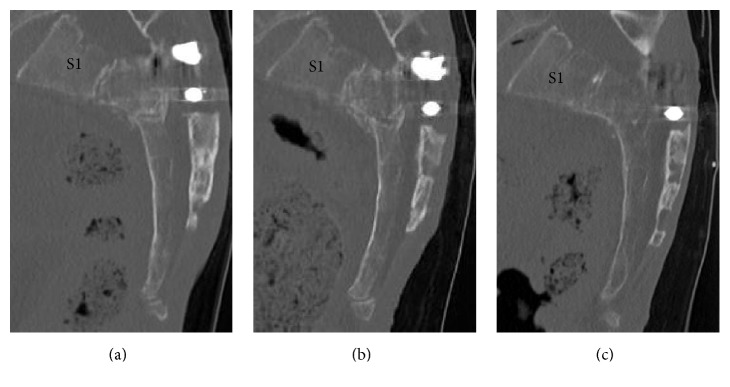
Follow-up sagittal reconstructed computed tomography (CT) images of the sacrum*. (a) One-month sagittal reconstructed CT image. (b) Three-month sagittal reconstructed CT image. (c) One-year sagittal reconstructed CT image. The second-third sacral vertebral body exhibited a high-density area (a). The high-density area in the second-third sacral vertebral body increased (b). The second-third sacral vertebral body had united without any displacement (c).
